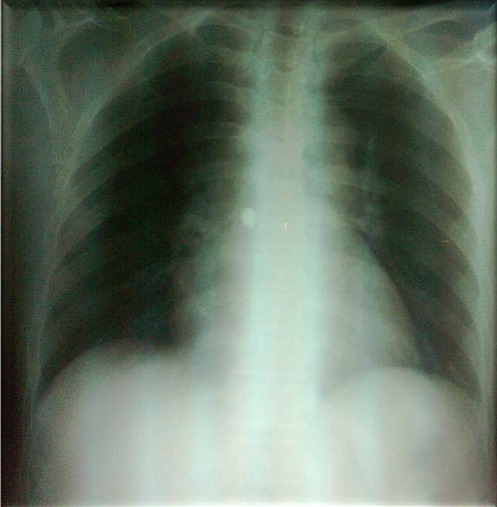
Chest X-ray showing Mediastinal enlargement
